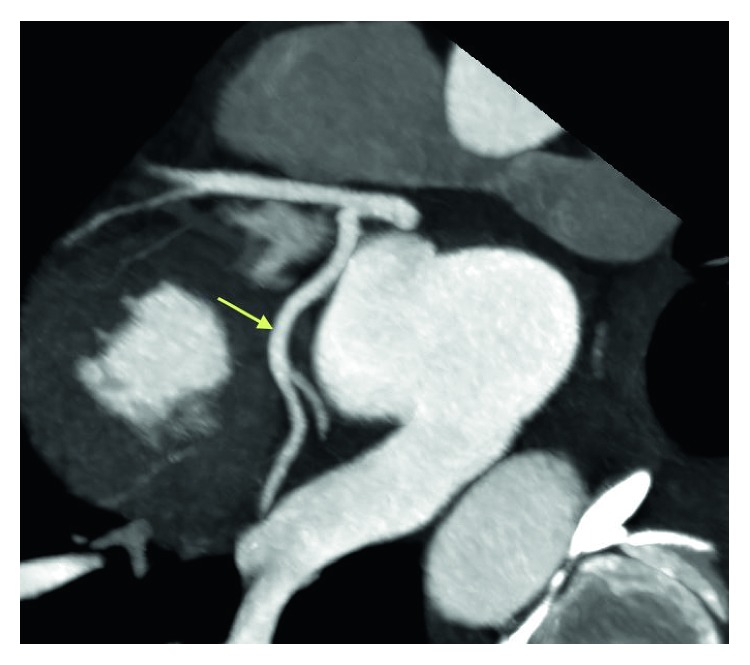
Coronary CT angiogram image revealing the circumflex is nondominant. The proximal segment and obtuse marginal branches are normal.
